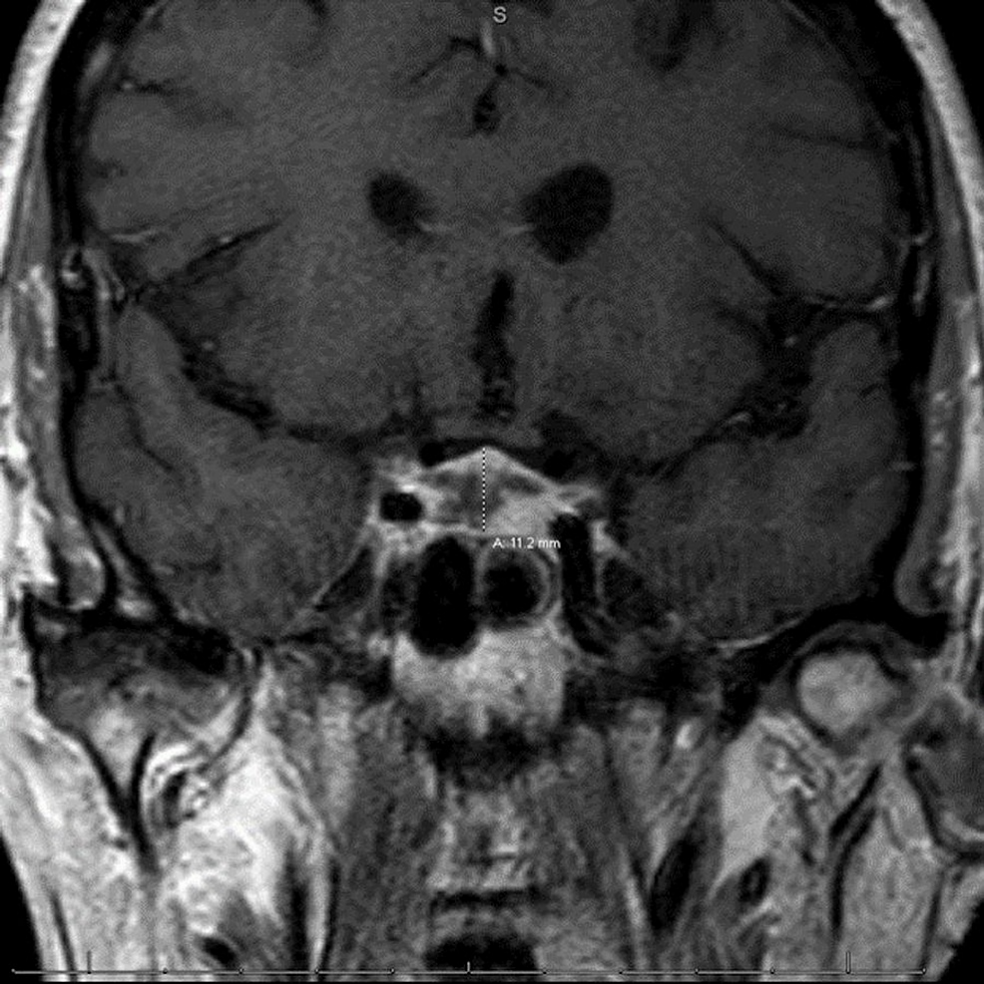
MRI of the brain with contrast T1 weighted coronal cross section highlighting abnormal enhancement and thickening demonstrating inflammation of the pituitary gland and stalk
